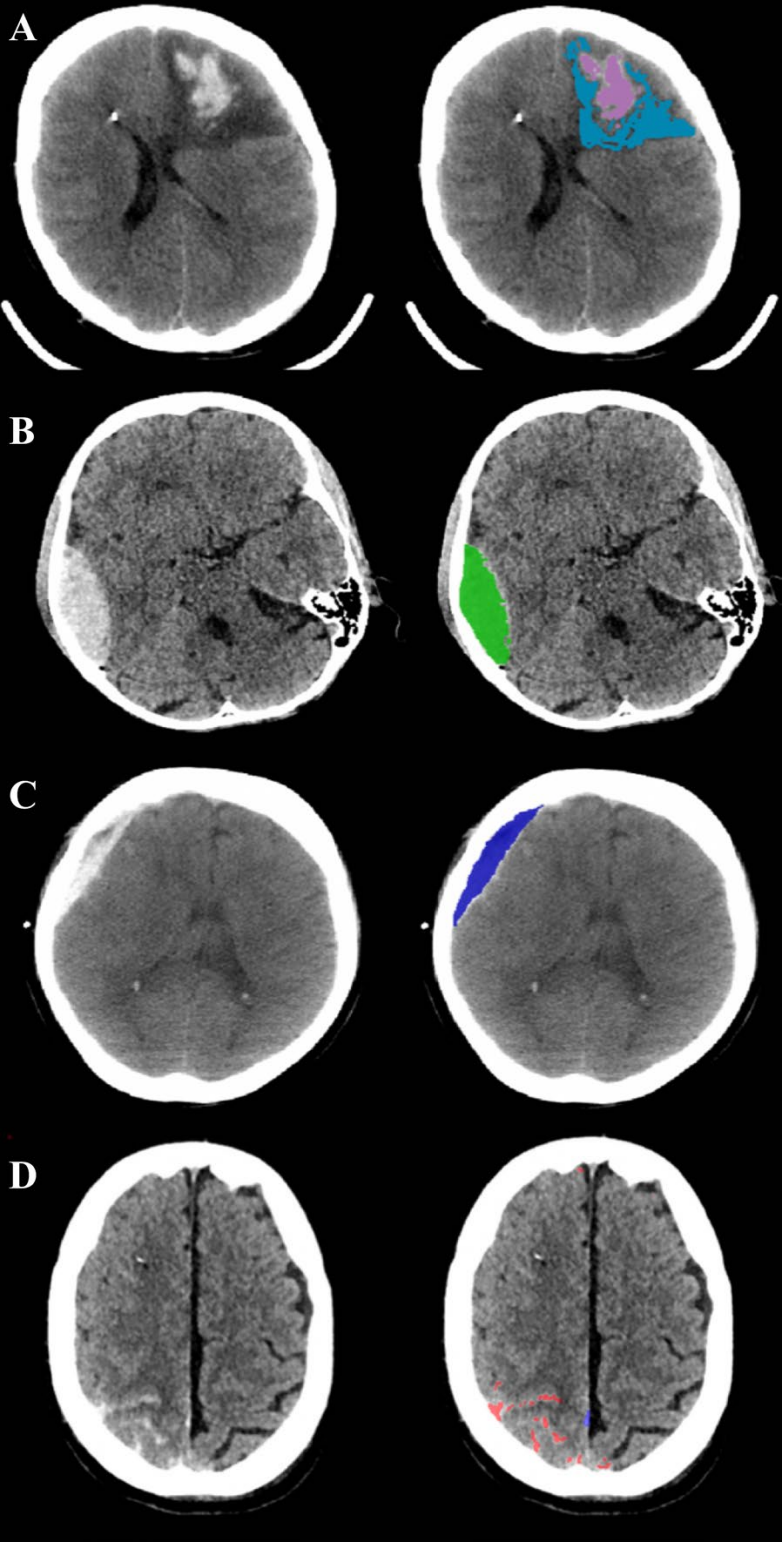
Representative set of segmented traumatic lesions on CT. Left: initial image; Right: segmented lesion(s). a Contusion core (blue) and peri-contusional edema (magenta), b epidural hematoma (green), c subdural hematoma (dark blue), d subarachnoid hemorrhage (red) and trace parafalcine extra-axial blood (dark blue). Right frontal hyperdensity in a and d represents the tip of the intracranial probe
